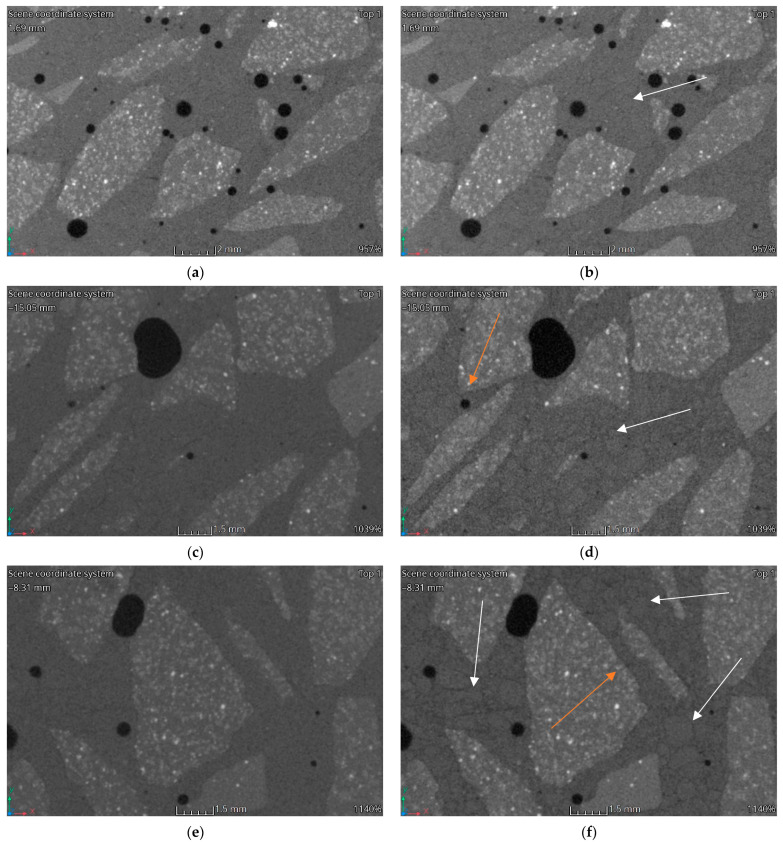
CT imaging results. Structural changes in three samples HPFRC 1.1: (a) 20 °C; (b) 200 °C; (c) 20 °C; (d) 400 °C; (e) 20 °C; (f) 600 °C.
